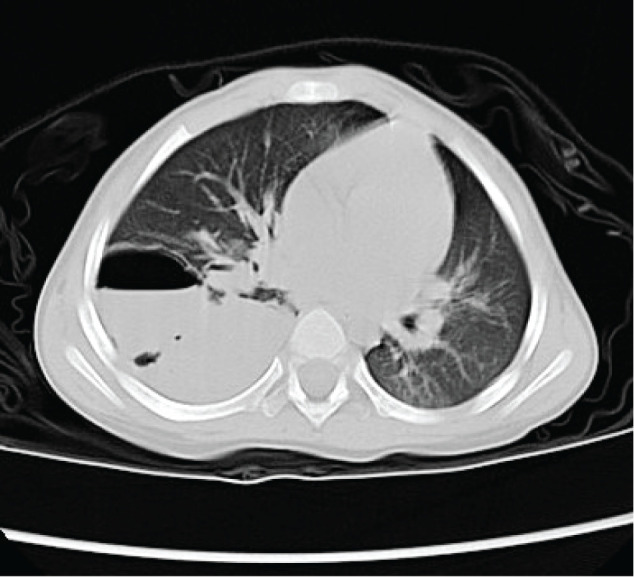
CT image of the right lower lobe abscess at the age of 1 year.
